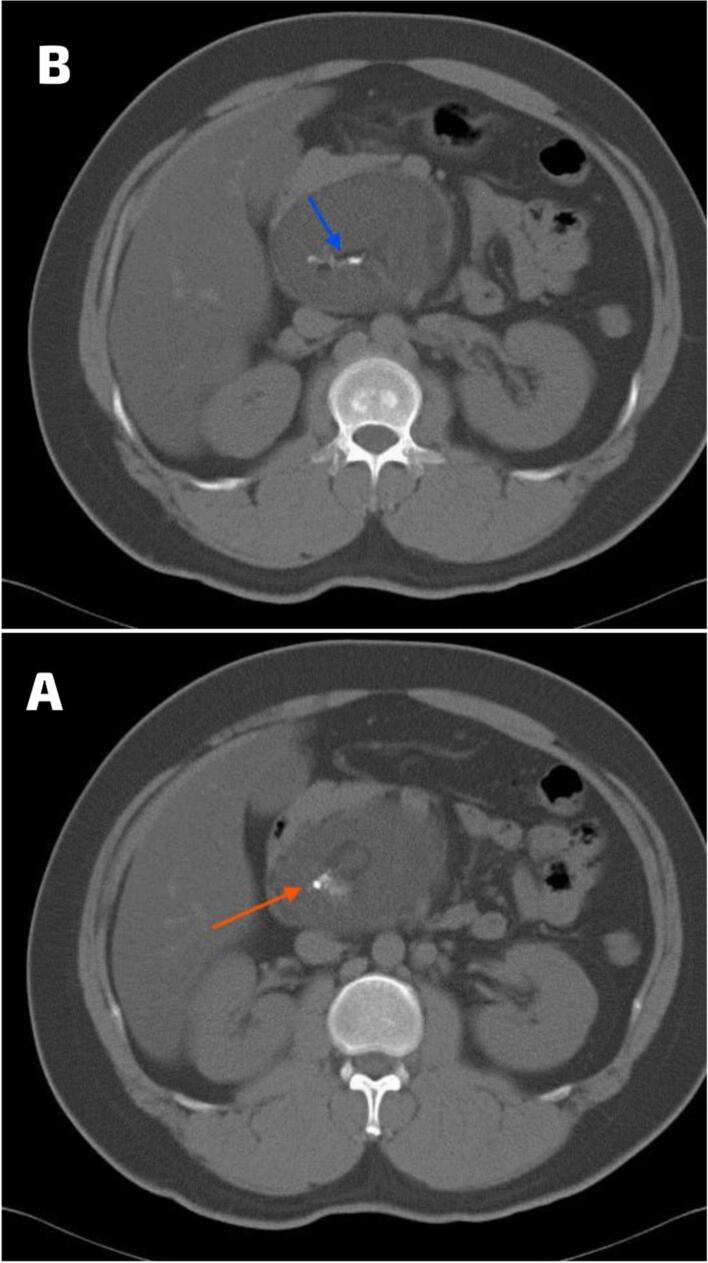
The axial CT scan without IV contrast revealed: A. multiple calcified areas at the center (red arrow); and B. a small area of fat density (blue arrow). (For interpretation of the references to colour in this figure legend, the reader is referred to the web version of this article.)
